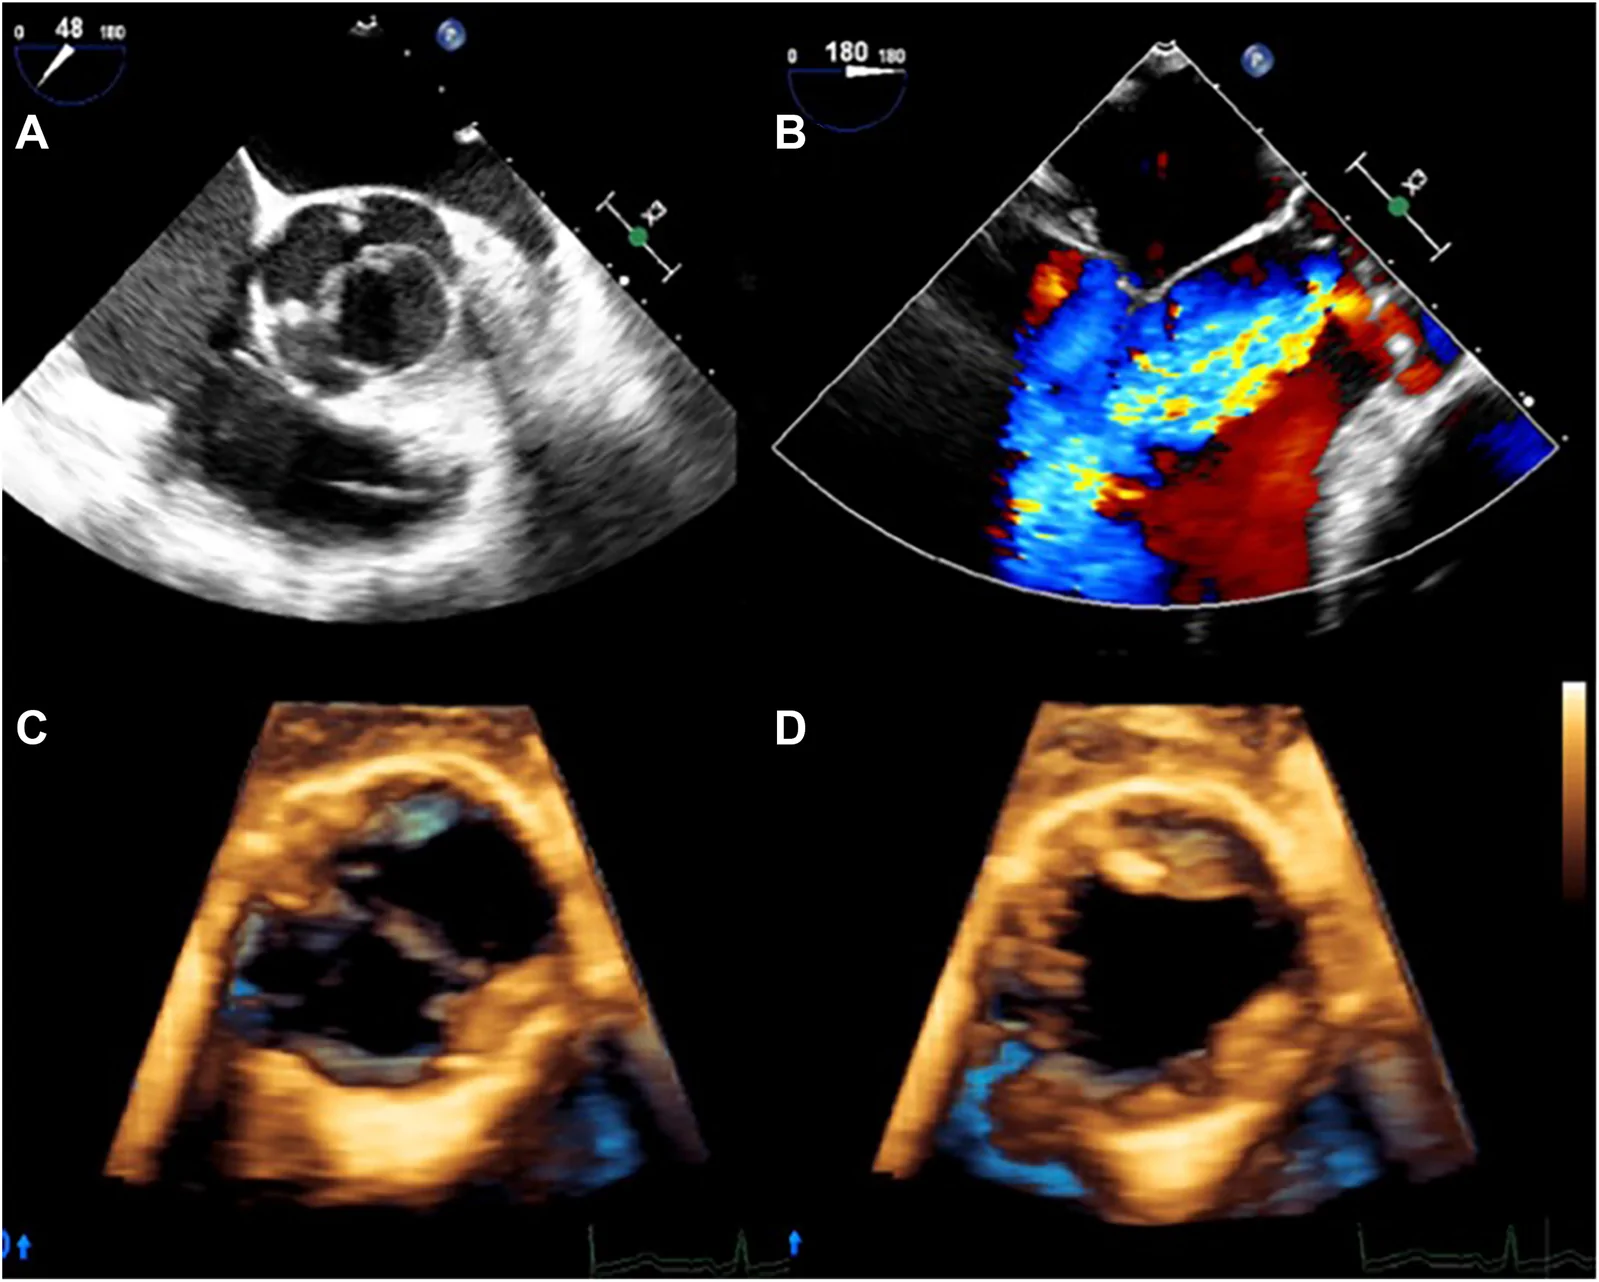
Preoperative transesophageal echocardiography, (A) short-axis and (B) long-axis views. Postoperative transesophageal echocardiography revealed satisfactory (C) valve opening and (D) closure.
